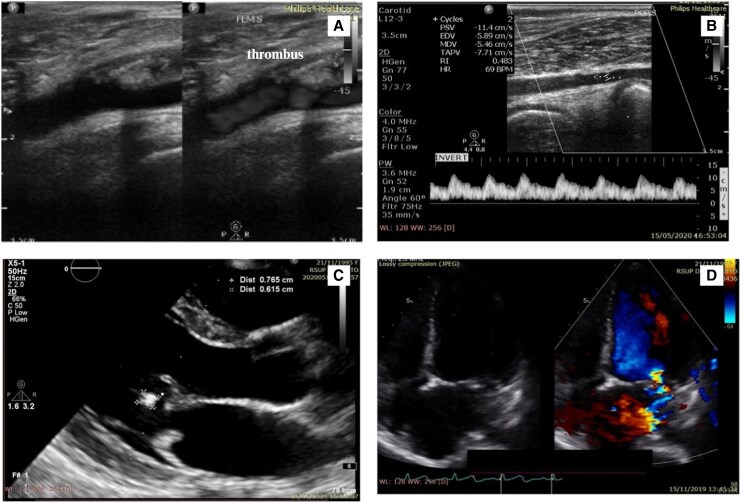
(A)* Arterial ultrasound showed thrombus at left femoral artery. *(B)* Monophasic Doppler wave at left popliteal artery. *(C)* Echocardiography showed vegetation on anterior mitral leaflet. *(D)* Mitral regurgitation with left atrium dilatation and concentric left ventricle hypertrophy.
